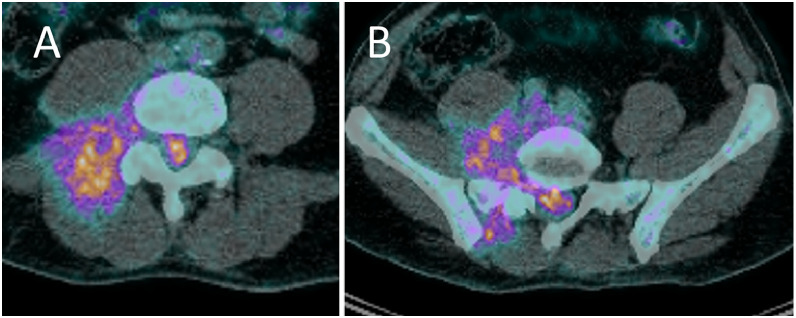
PET-CT image at the lower lumbar level (A) and upper sacral level (B) showing increased ^18^F-FDG uptake within the spinal canal, around the right L5 nerve root, around the right paravertebral muscle, as well as the right psoas and erector spinae muscles, corresponding to the location of the tumoural mass.
